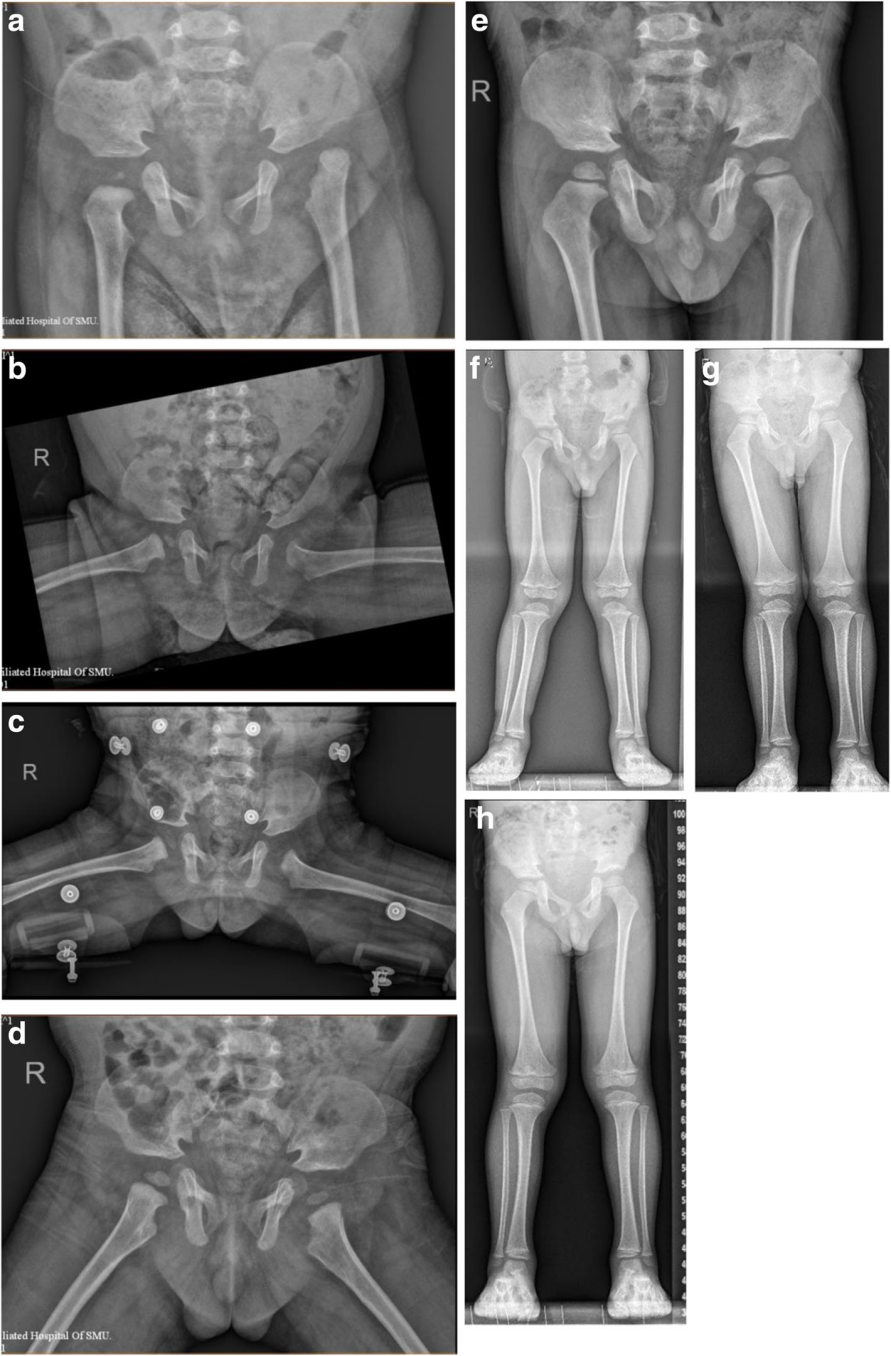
X-ray images of one patient before the operation and during follow-up. a Pre-operational imaging. b Arthroscopic reduction (concentric reduction) at 1 day after the operation, c 3 months of follow-up, d 12 months of follow-up, e 2 years of follow-up, f 3 years of follow-up, g 4 years of follow-up, and h 5 years of follow-up
